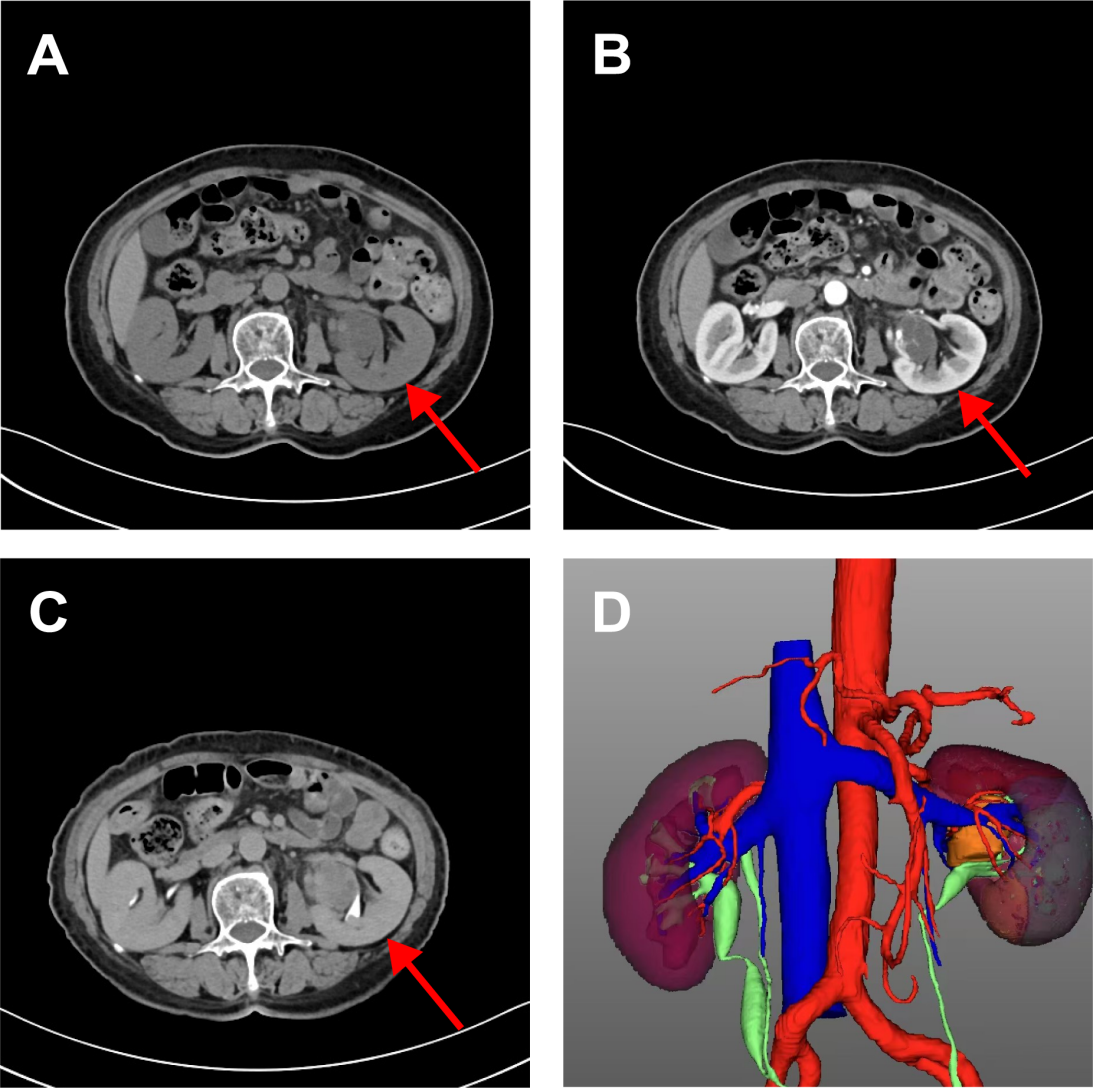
CT urography imaging of renal anastomosing hemangioma. Flat sweep (A) enhanced cortical phase; (B) enhancing draining phase; (C) and reconstructed three-dimensional images (D). It showed a cystic solid space-occupying lesion adjacent to the left renal pelvis measuring 3.4 cm*2.6 cm. It is poorly demarcated from the adjacent ureter and is considered a possible low-grade malignant neoplastic lesion. The reconstructed three-dimensional clearly showed the lesion and surrounding anatomical structure.
